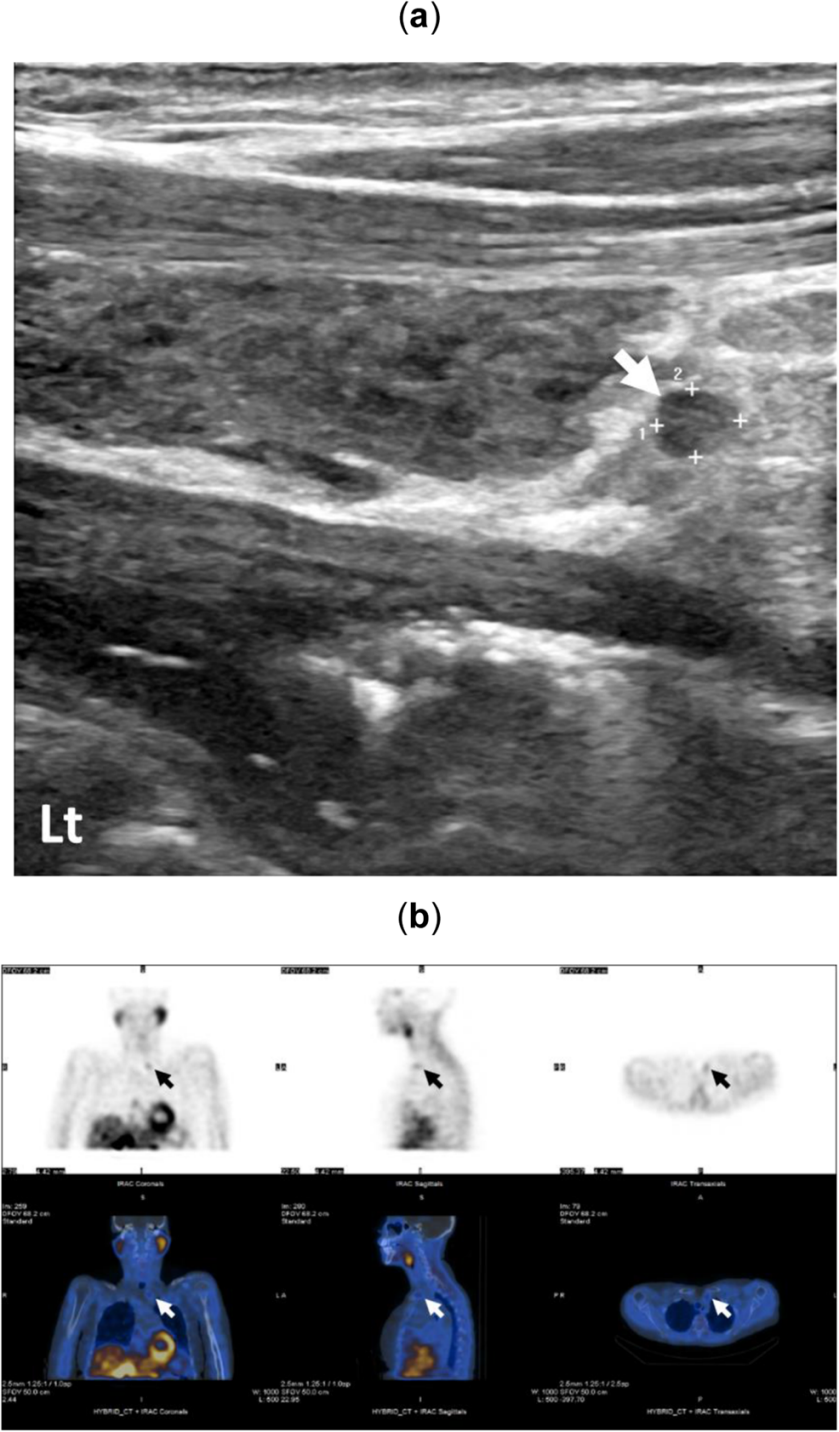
Parathyroid adenoma identified by ultrasound scan measuring 2.6 × 2.1 mm at the inferior aspect of the left thyroid lobe (a). Anterior maximum intensity projection images of SPECT showing delayed washout of radiotracer (b) and delayed-phase coronal, sagittal and axial fused ^99m^Tc-Sestambi-SPECT/CT images showing a parathyroid adenoma localising posterior to the inferior pole of the left lobe of thyroid (c) of a woman (case 1)
